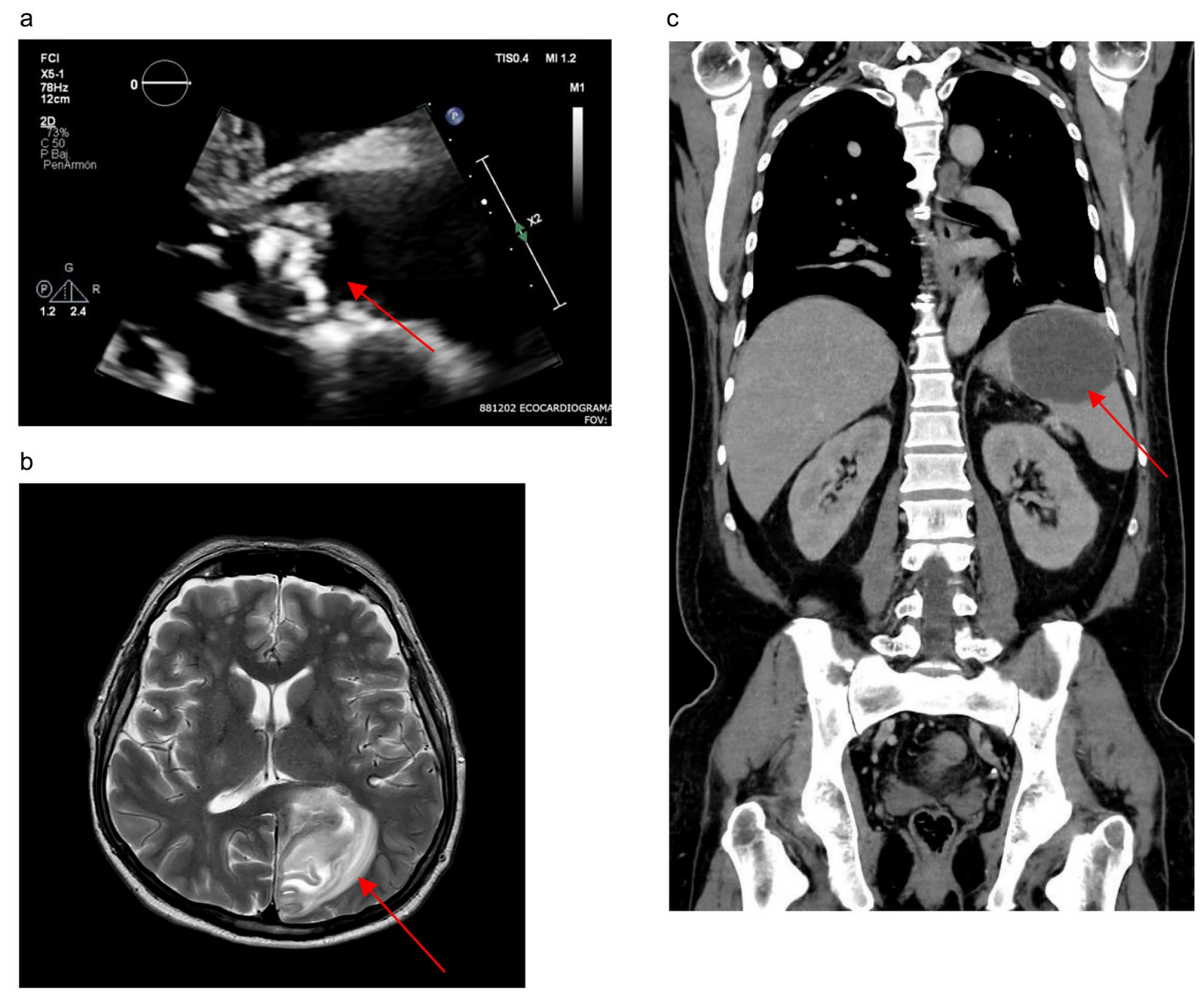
Imaging studies. (a) Transthoracic echocardiogram, showing a large vegetation of the aortic valve (red arrow). (b) Transverse view of brain MRI, showing late subacute infarction with small left occipital hemorrhagic transformation (red arrow). (c) Coronal view of abdominal CT, showing a large unilocular splenic abscess (red arrow)
